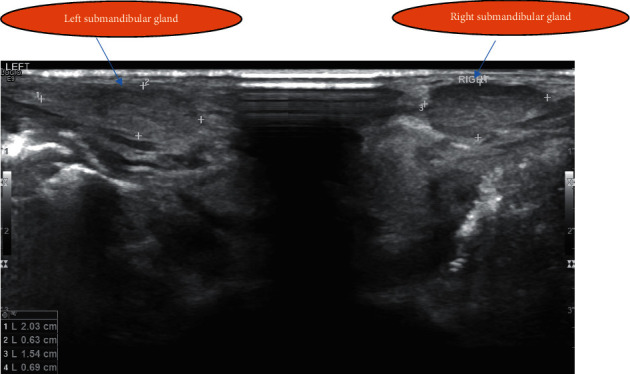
Ultrasound with bilateral submandibular gland enlargement (hypoechoic area).
